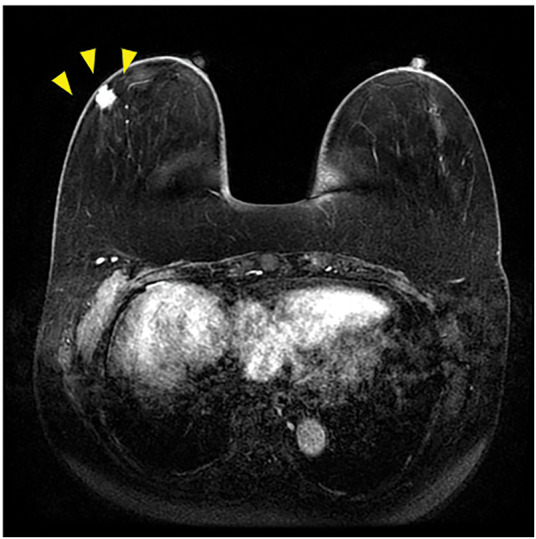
Gadolinium-enhanced MRI image. A 1.2-cm spiculated mass in contact with the skin was found in the D region of the right breast. The wedge markers indicate the lesion.
